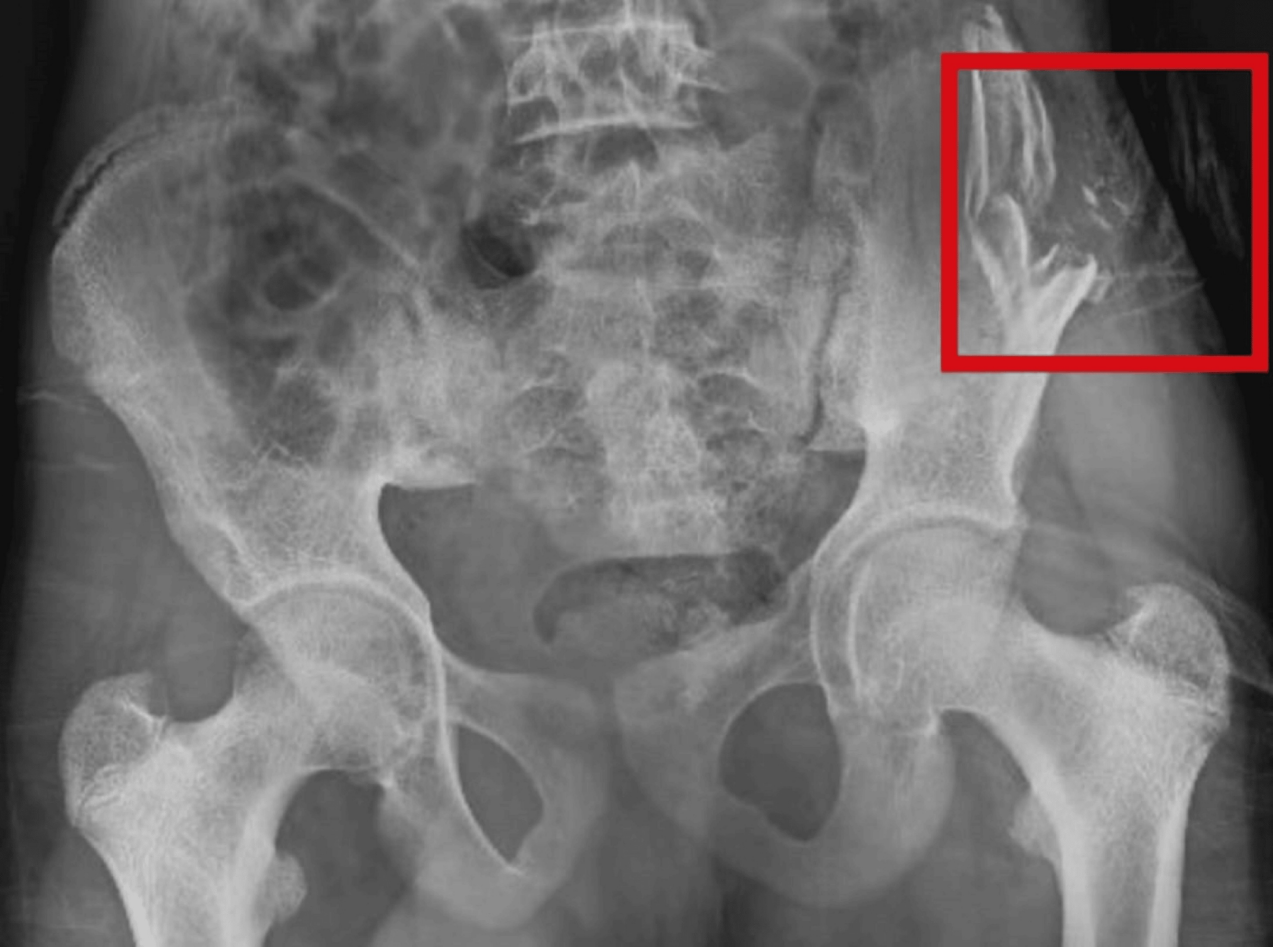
Radiographic scan, in which the red box marking shows compound grade 3B iliac blade fracture
